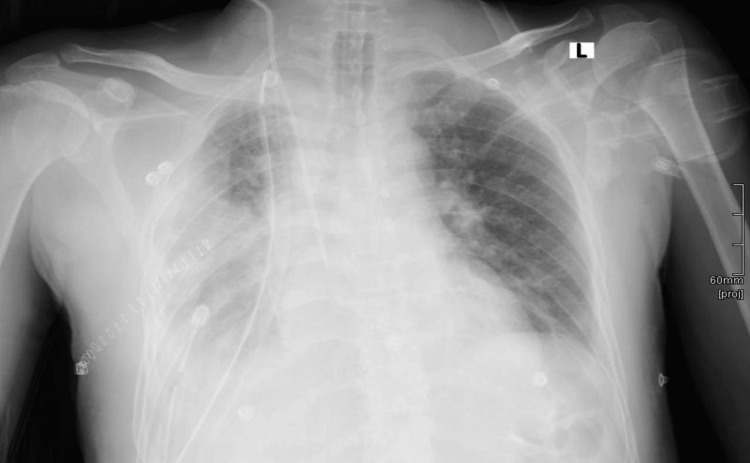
Chest X-ray showed a massive pleural effusion on the right side.
